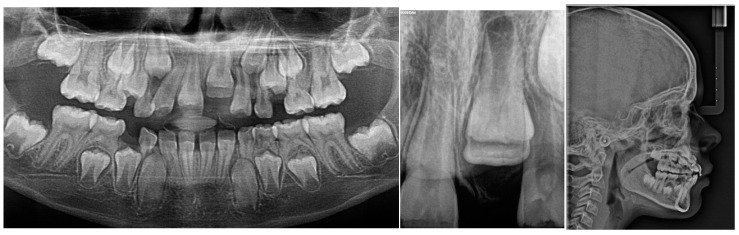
Radiography in April 2024.
